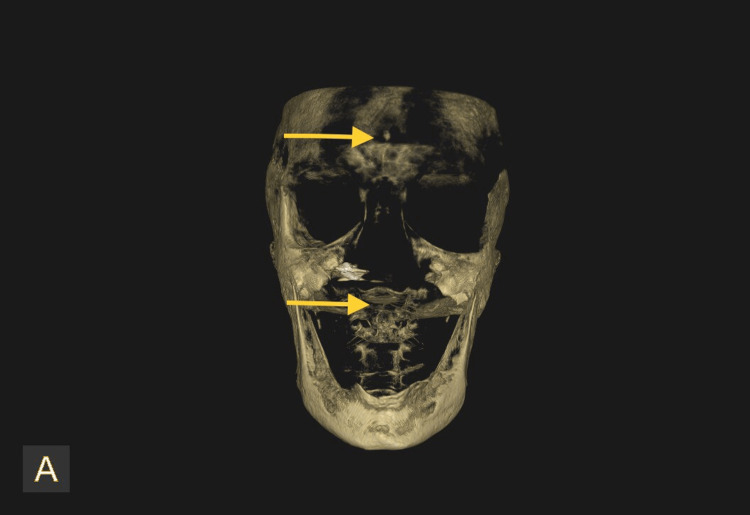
Three-dimensional view of CBCT after eight months Three-dimensional views of CBCT taken after eight months shows the increased thickness of frontal bone, parietal bone, and maxilla with obliteration of marrow spaces, alteration of trabecular pattern with cotton wool/ground glass appearance. Reduced maxillary sinus space is also evident.
